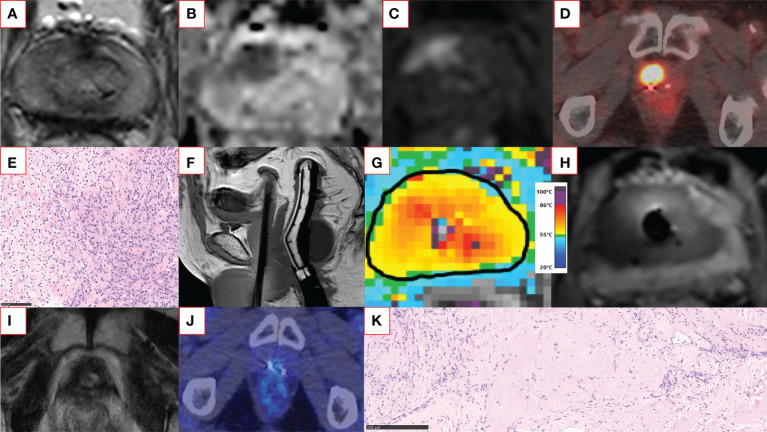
An example of a successful salvage TULSA patient case. The patient had a rising PSA of up to 13 ng/ml within six years after primary external beam radiotherapy. Screening T2-weighted (A) and diffusion-weighted (B) MRI showed a distinct anterior lesion with early enhancement on gadolinium-enhanced imaging (C) graded as PI-RR 5 lesion. The same lesion was also clearly visible in ^18^F-PSMA-1007 PET-CT (maximum standardized uptake value 23) (D). Two residual gold fiducial markers implanted before image-guided radiotherapy are also visible next to the PSMA-positive lesion (D). The MRI-targeted biopsy from the lesion revealed vital carcinoma resembling ISUP GG 5 disease (E). The patient underwent whole-gland TULSA. On the sagittal T1-weighted image (F), a transurethrally inserted ultrasound applicator, endorectal cooling device, and suprapubic catheter are in place. The targeted region reached a lethal minimum temperature of 55°C (G). The non-perfused volume can be visualized immediately after treatment, demonstrating the acute ablation effect covering the targeted lesion (H). At 12 months, the patient underwent follow-up imaging with multiparametric MRI and ^18^F-PSMA-1007 PET-CT (I, J), both negative for cancer. The prostate volume decreased from 20 cm^3^ to less than 1 cm^3^ at 12 months. The 12-month post-TULSA biopsy agreed with imaging findings and showed only a treatment effect with no signs of cancer (K). At the recent follow-up visit two years after TULSA, PSA is still low (PSA 0.067 ng/ml) and stable, and the patient has leak- and pad-free continence and erections sufficient for intercourse. The TULSA treatment report of the patient case, including treatment planning, thermal mapping, and post-treatment gadolinium-enhanced images, is provided in Supplementary Figure S1. CT, computed tomography; ISUP GG, International Society of Urological Pathology grade group; MRI, magnetic resonance imaging; PET, positron emission tomography; PI-RR, Prostate Imaging for Recurrence Reporting; PSA, prostate-specific antigen; PSMA, prostate-specific membrane antigen; TRUS, transrectal ultrasound; TULSA, Transurethral ultrasound ablation.
